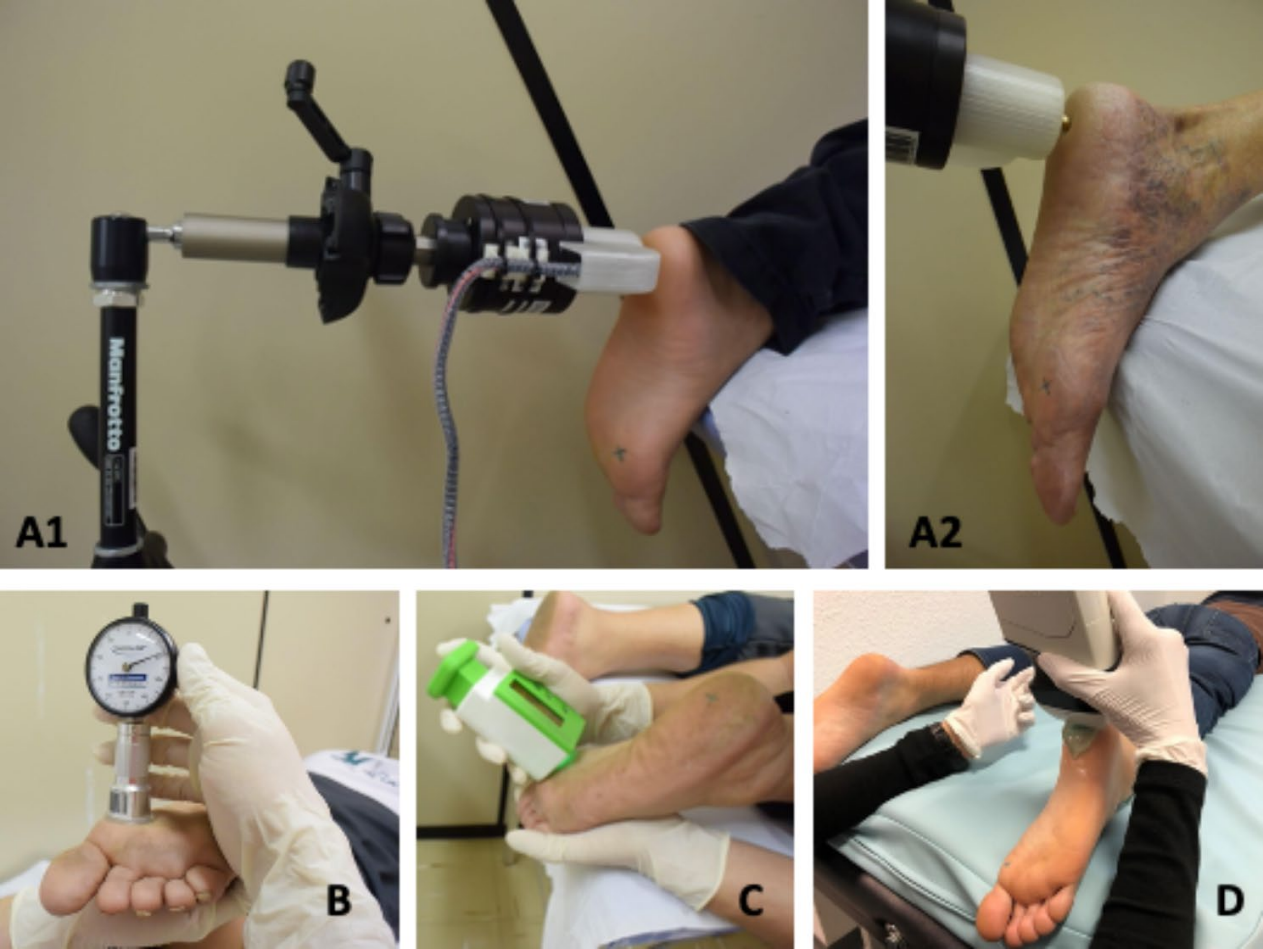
Measurement of the mechanical properties of plantar skin and superficial soft tissue. A1 and A2: Modified vibration exciter (Mini-Shaker); B: Custom-built indentometer; C: Shore 00 Durometer; D: Handheld ultrasound device
